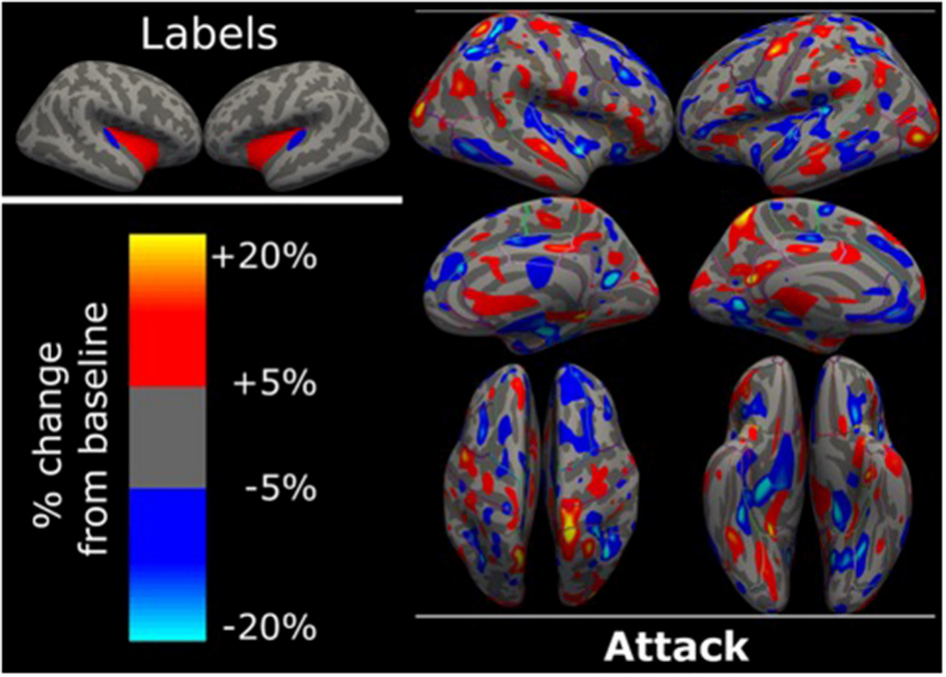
Surface based analysis. Surface based analysis in FreeSurfer showing percentage changes of ^18^F-FDG uptake during MD attack compared to the patient's own baseline ^18^F-FDG scan. There were no significant changes of more than 20% change between the ^18^F-FDG uptake during MD attack compared to the patient's own baseline scan obtain 7 days later. Labels for anatomical orientation are shown in the upper left corner. Blue is Heschl's gyrus, red is insula, as defined by the Desikan-Killeany atlas. Dark gray is sulci, light gray is gyri.
